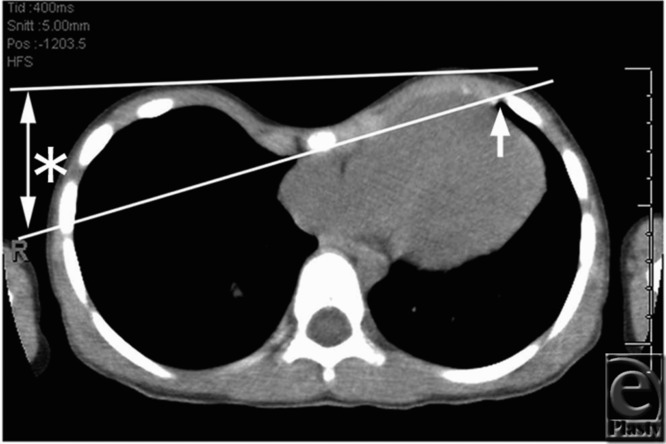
A 9-year-old boy with symmetric chest wall deformity. The insertion point for the dissector was determined by CT examination. The insertion point of the Nuss bar on the left thoracic wall (↑). The depth between the body surface and the insertion point (*) was 7.5 cm.
